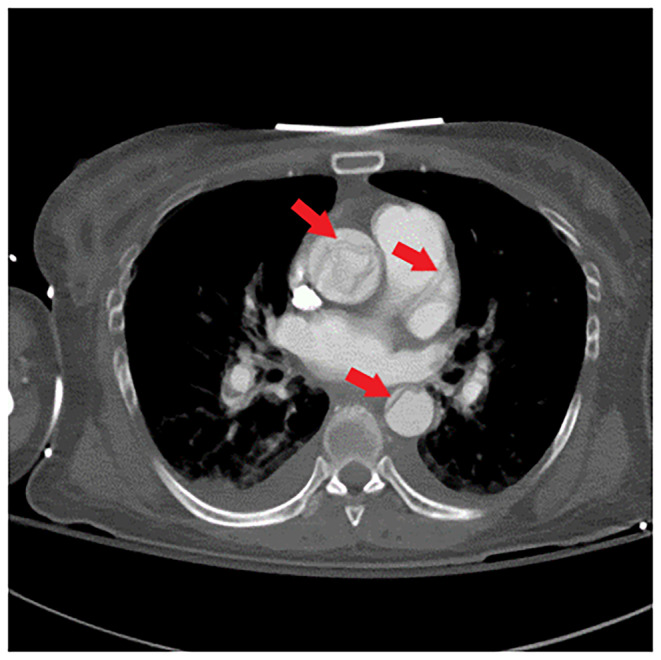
Axial image of CTA chest demonstrates type A dissection flap involving the thoracic aorta (red arrows), which extends inferiorly to the level of the aortic valve. Dissection flaps extend into the proximal portions of all three arch vessels, with more superior extension into the left common carotid artery.
